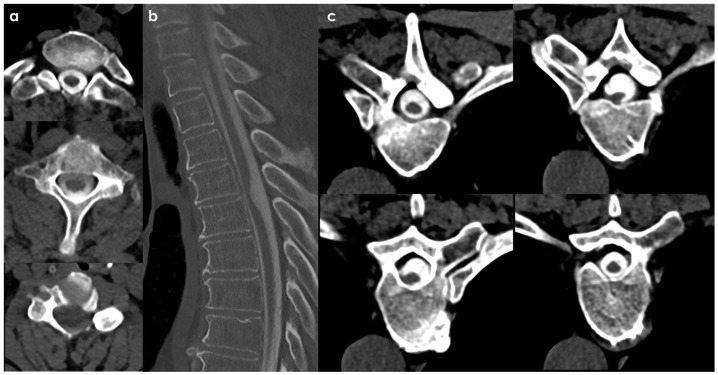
CT Myelography of the same patient as Figure 6, showing axial supine (a), sagittal (b) and axial prone (c) images, showing the eccentric attraction of the spinal cord at the level of the intervertebral discus.
